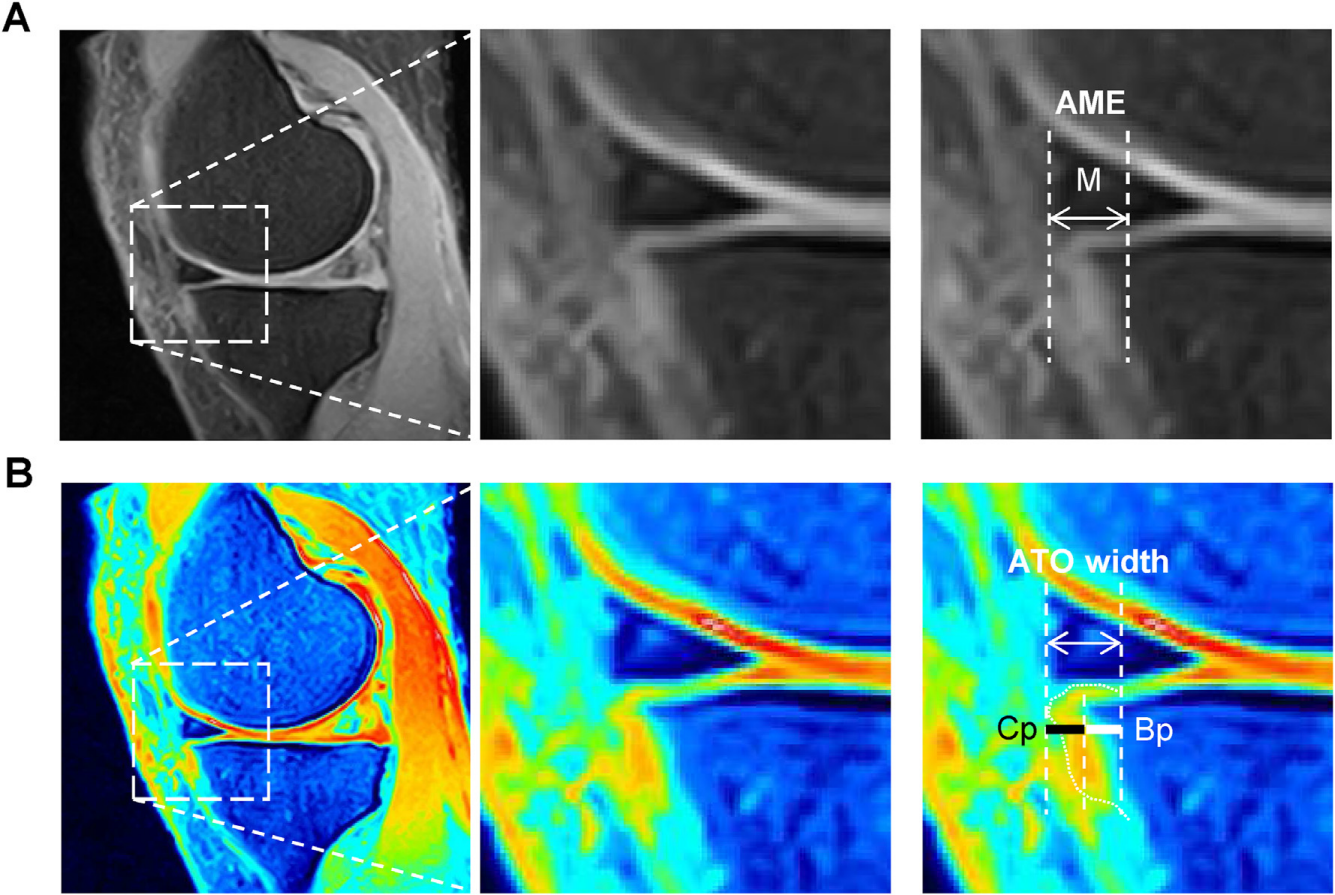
Representative data on the measurement of AME and ATO width by PDFS and PPDFS MRI images of the knee joint, respectively. (A), Measurement of AME on the sagittal PDFS image. AME (white horizontal line with arrows) was measured as the distance between the vertical dotted line drawn at the outer edge of the medial meniscus and the vertical dotted line drawn at border of the tibia, making the rectangle-shaped anterior edge of the tibia appear. (B), Measurement of ATO width using PPDFS image. PPDFS view was prepared by pseudo-coloring the PDFS MRI image as described in the Methods. Vertical dotted lines indicate the anterior edge of the tibia excluding osteophyte, the boundary between the bone part of osteophyte (Bp) (white horizontal line) and cartilage part of osteophyte (Cp) (black horizontal line), and the outer edge of the cartilage part of osteophyte. White horizontal line with arrows indicates full-length (Bp plus Cp) width of ATO. PDFS, proton density-weighted fat-suppressed; PPDFS, pseudo-colored PDFS; M, medial meniscus; AME, anterior meniscus extrusion; ATO, anterior tibial osteophyte.
